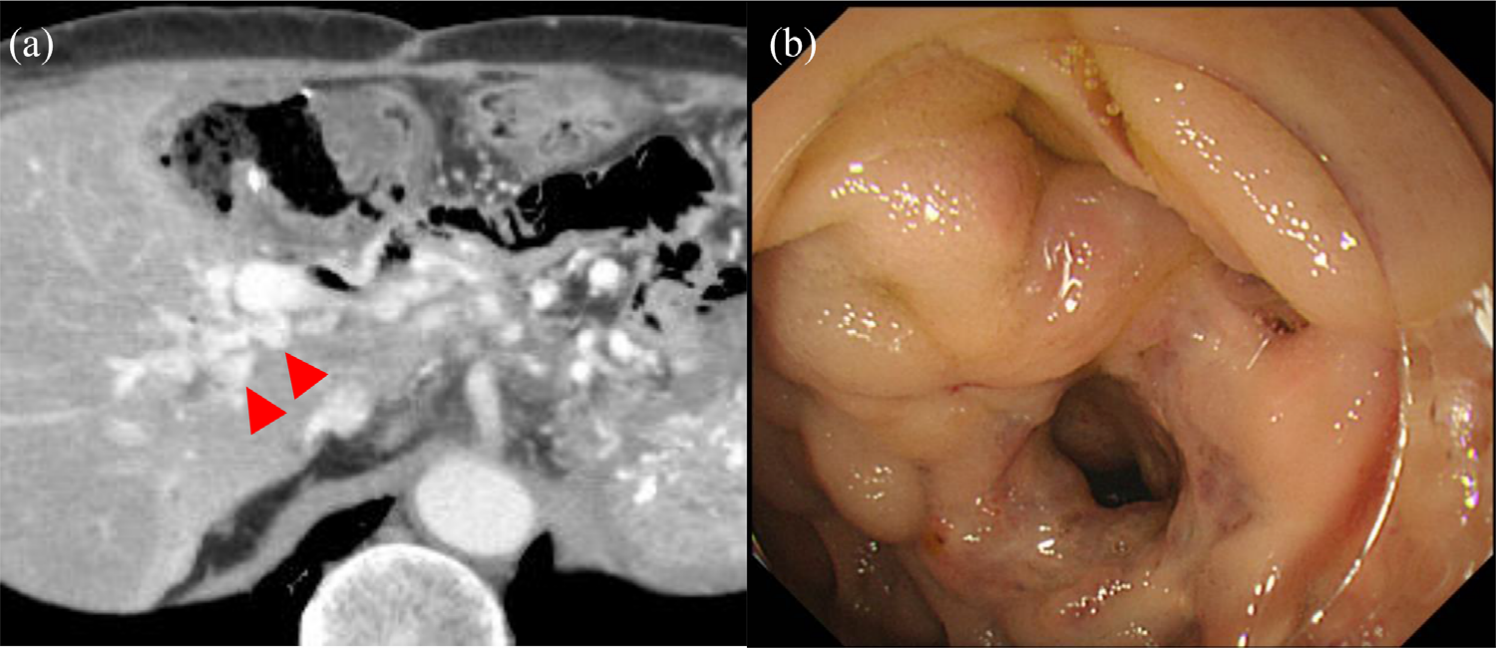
Images of case 1 before treatment: (a) Contrast‐enhanced computed tomography showed dilated veins around the choledochojejunostomy. (b) Ectopic varices around the choledochojejunostomy were observed by endoscopy
